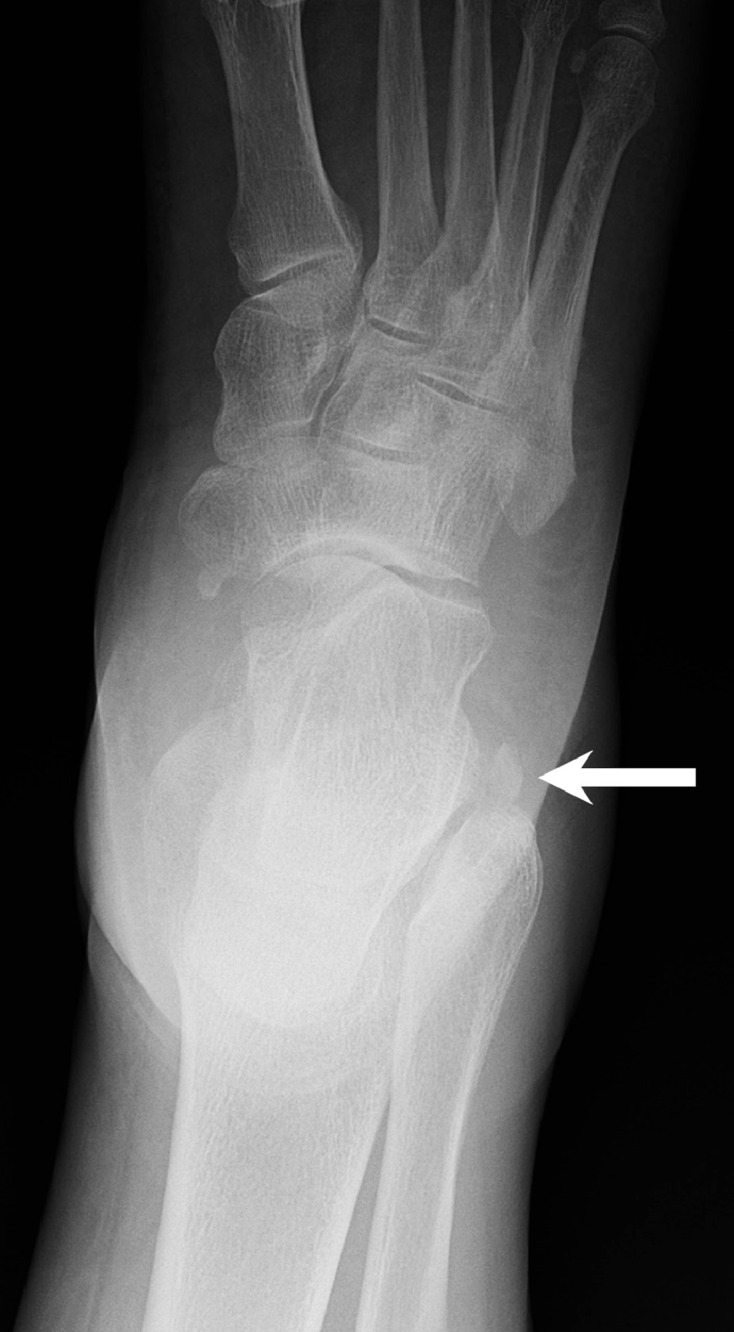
AP radiograph of the right foot 12 days after initial presentation demonstrates proximal migration of the os peroneum to the level of the lateral talus (arrow), suggesting injury to the IPR but an intact SPR.
